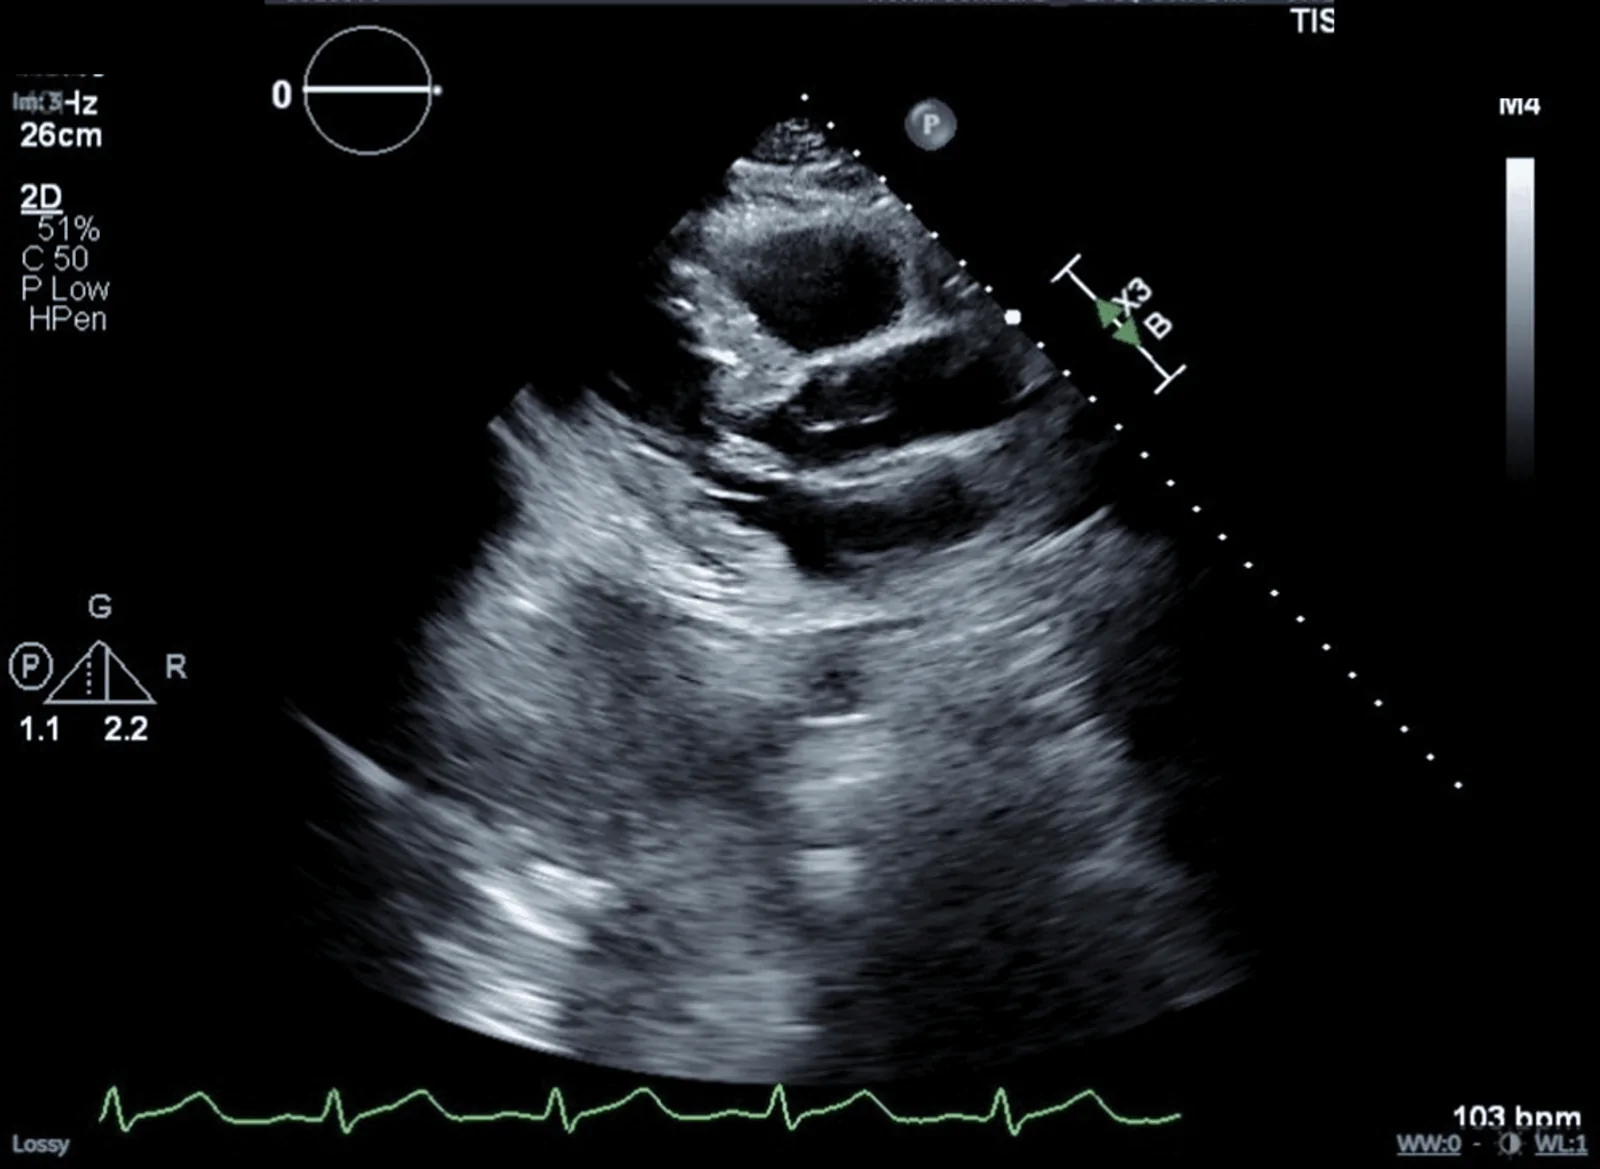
Transthoracic echocardiographic image Echocardiography demonstrated mild left ventricular hypertrophy with preserved left ventricular systolic function (LVEF approximately 60%) and normal wall motion. Right ventricular systolic function was preserved, with no pulmonary hypertension or pericardial effusion. LVEF: left ventricular ejection fraction
